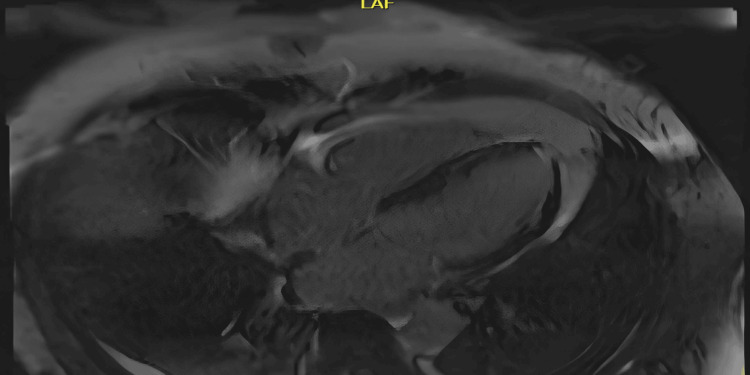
Cardiac MRI four-chamber phase-sensitive inversion recovery sequence demonstrating multifocal patchy late gadolinium enhancement due to inflammation/scarring in the mid-wall and subepicardial wall of the left ventricle involving the interventricular septum, inferolateral wall at the mid and apical level, and the true apex as well as the free wall of the right ventricle concerning for acute myocarditis MRI: magnetic resonance imaging
